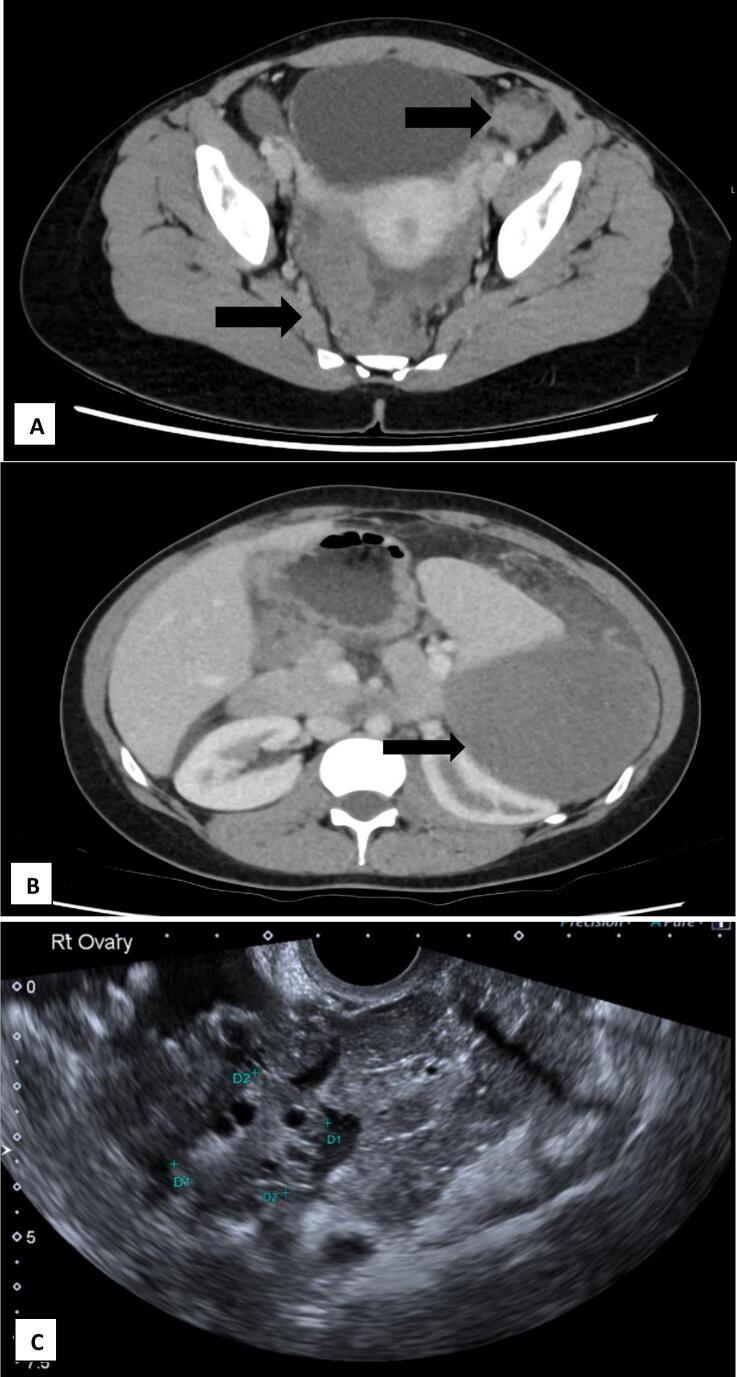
A- Axial view of pelvic CT scan demonstrating peritoneal nodules in the Pouch of Douglas, left lower quadrant nodular disease, ascites. B- Axial view of abdominal CT scan demonstrating large peritoneal mass in the left upper quadrant compressing the spleen and kidney. C- Sonographically normal right ovary surrounded by peritoneal nodules.
